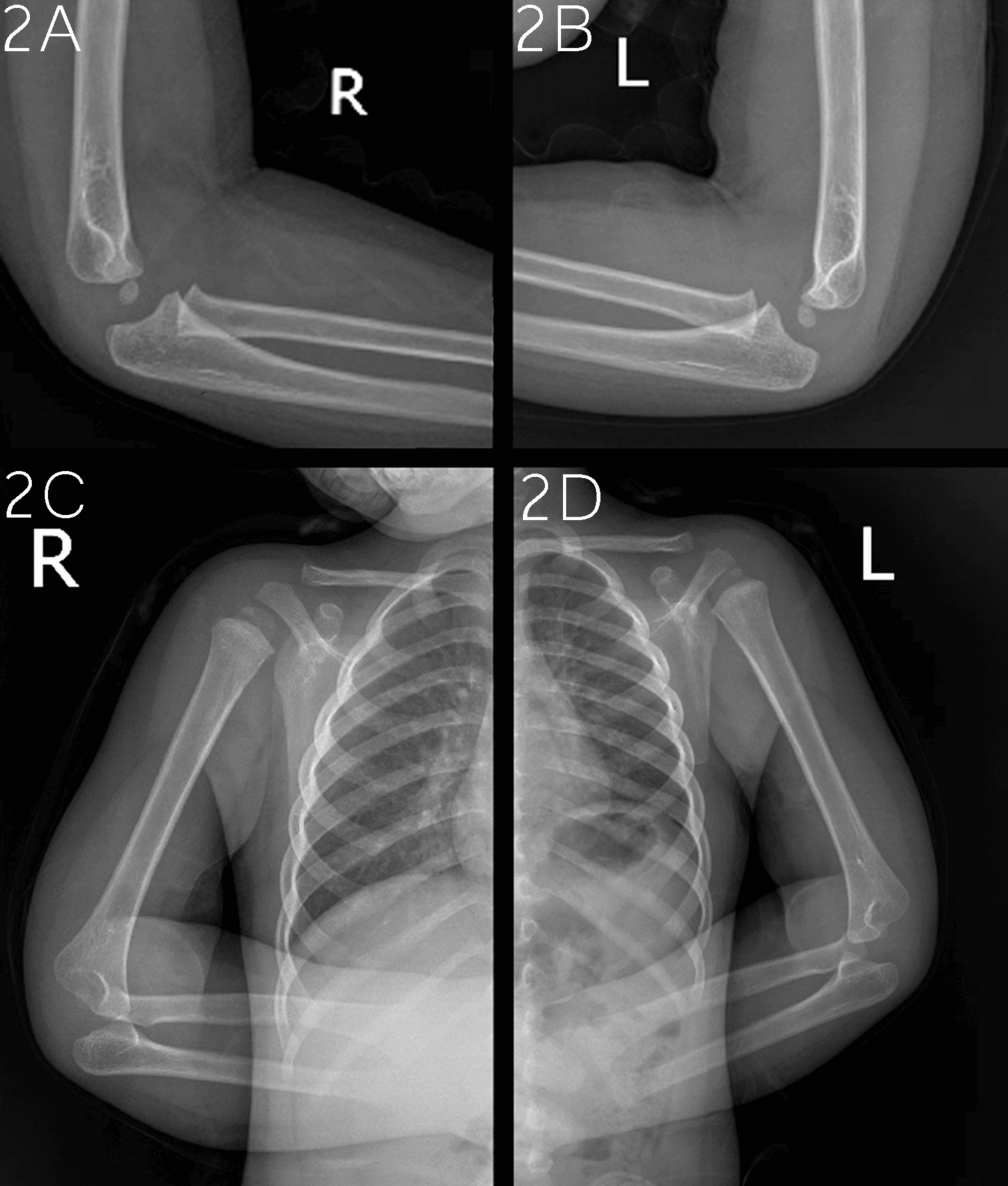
Elbows and humeri plain radiographs. Right elbow lateral view (2A, top left); Left elbow lateral view (2B, top right); Right humerus lateral view (2C, bottom left); Left humerus lateral view (2D, bottom right).
